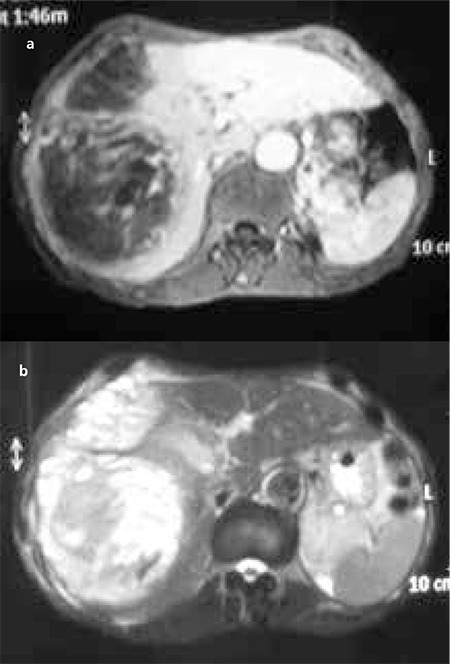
Magnetic resonance imaging. There was a large mass (152x120x135 mm) with heterogeneously hypointense on the T1-weighted image (a), and heterogeneously hyperintense on the T2-weighted image. After the administration of contrast material, heterogeneous hyperenhancement was observed in the solid parts of the lesion (b). This mass covered segment 7-8 completely and segment 4 partially.
